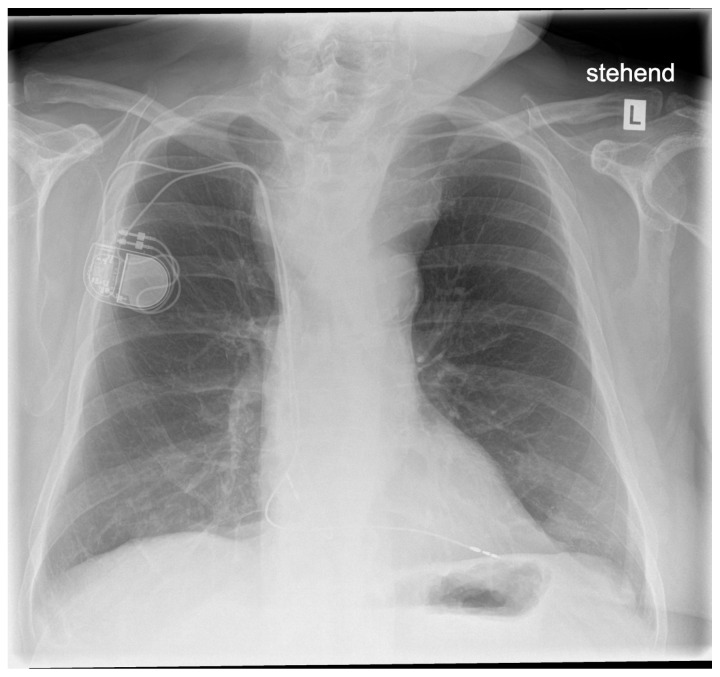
Preoperative chest X-ray with prominent tracheal deviation.
